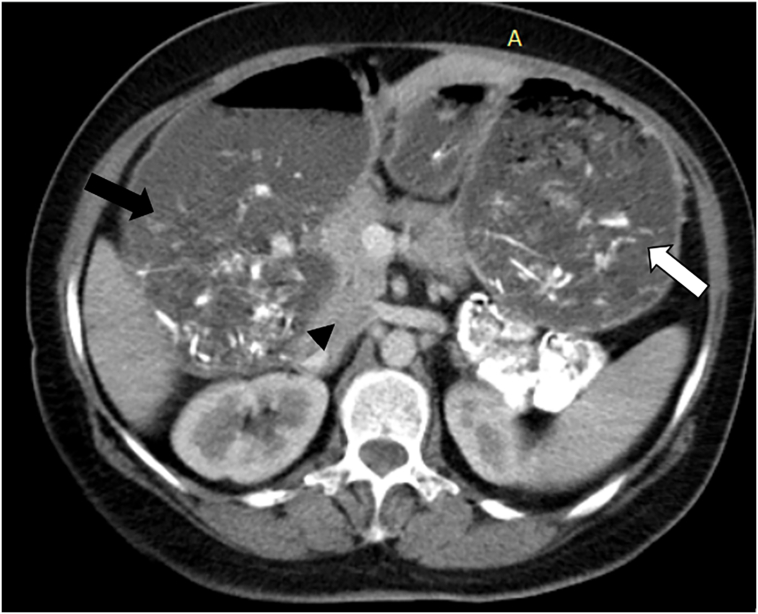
Contrast-enhanced CT images (axial plane) showing a dilation in stomach (white arrow), a dilation of the first part of the duodenum (black arrow) and the head of pancreas (arrowhead).
